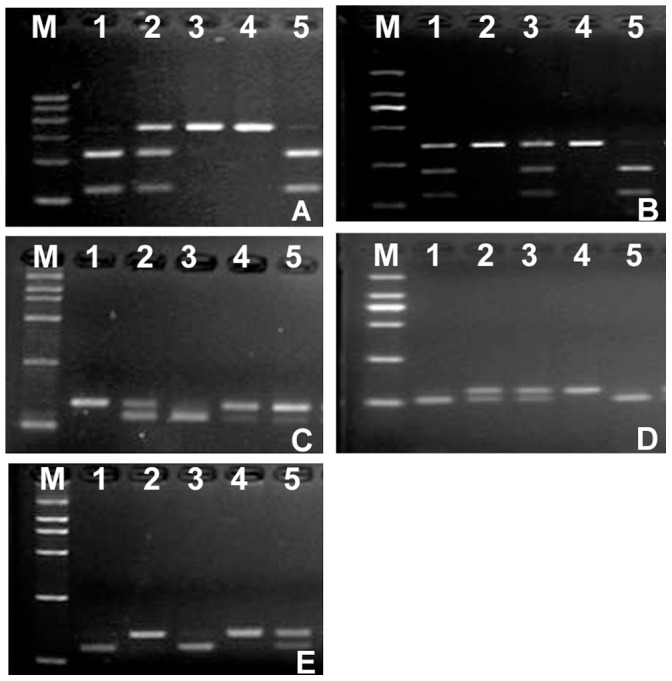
Example of the differrent genotype of five SNPs. (A) rs3093024: laneM, molecular size standard; lane 1,5, genotype GG(217 bp and 123 bp); lane 2, genotype AG(340 bp, 217 bp and 123 bp); and lane 3,4, genoty (340 bp). (B) rs6902119: lane 1,3, genotype CT(366 bp, 228 bp and 138 bp); lane 2,4, genotype CC(366 bp); and lane 5, genotype TT(228 bp and 138 bp). (C) rs3093023: lane 1, genotype AA(114 bp); lane 2,4, 5, genotype AG(114 bp and 27 bp); and lane 3, genotype GG(27 bp). (D) rs968334: lane 1,5, genotype GG(20 bp); lane 2,3, genotype AG(100 bp and 20 bp); and lane 4, genotypeAA(100 bp). (E) rs2301436: lane 1,3, genotype GG(21 bp); lane 2,4, genotype AA(120 bp); and lane 5, genotype AG(120 bp and 21 bp).
